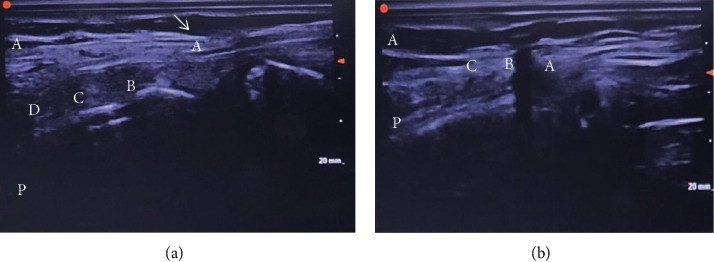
Needle localization on ultrasound longitudinal view. (a) (A) cricoid cartilage, (B) 1st tracheal ring, (C) 2nd tracheal ring, and (D) 3rd tracheal ring; (white arrow) needle black shadow. (A) Anterior side and (P) posterior side. (b) (A) cricoid cartilage, (B) 1^st^ tracheal ring, and (C) 2^nd^ tracheal ring; (white arrow) needle black shadow between (B) and (C). (A) Anterior side and (P) posterior side.
